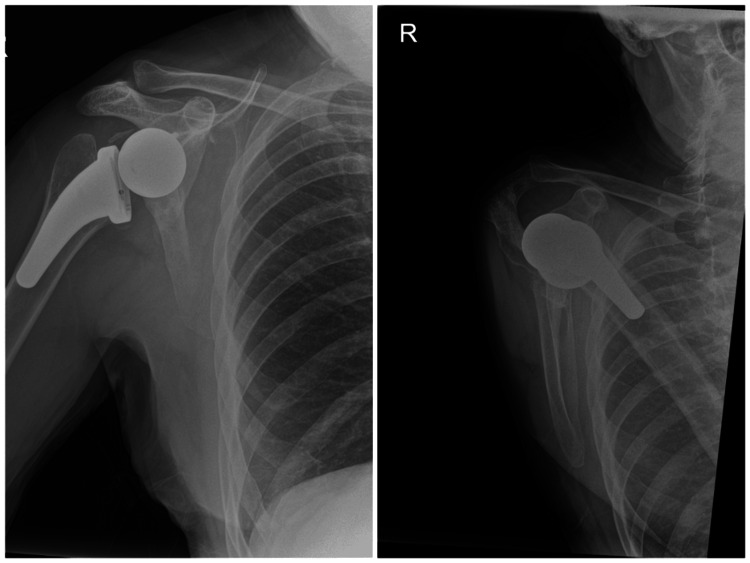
Day-one post-operative radiographs demonstrating well-positioned reverse shoulder arthroplasty with satisfactory implant alignment and stable fixation of the glenoid and humeral components.
